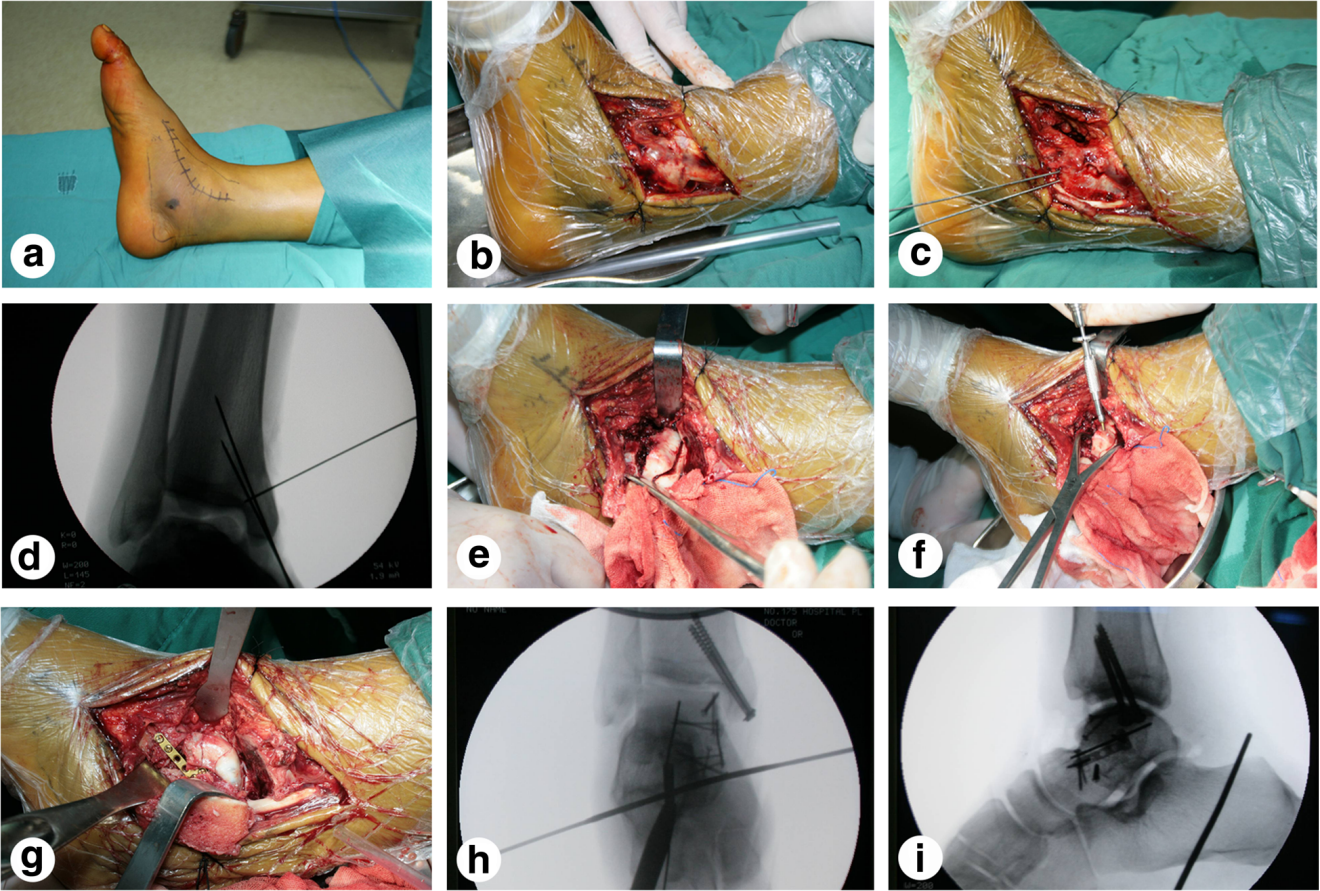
Surgical procedure through approach of medial malleolar osteotomy and mini-plate for fixation (case 3). a Arc skin incision. b Exposure of malleolus medialis. c Preparation for medial malleolar osteotomy. d X-ray showing kirschner wire in good position. e Medial malleolar osteotomy was performed. f–g Talar neck fracture fixed with mini-screws and mini-plate. h–i Anterioposterior and lateral view of X-ray showing the screws and plate appropriately fixed
